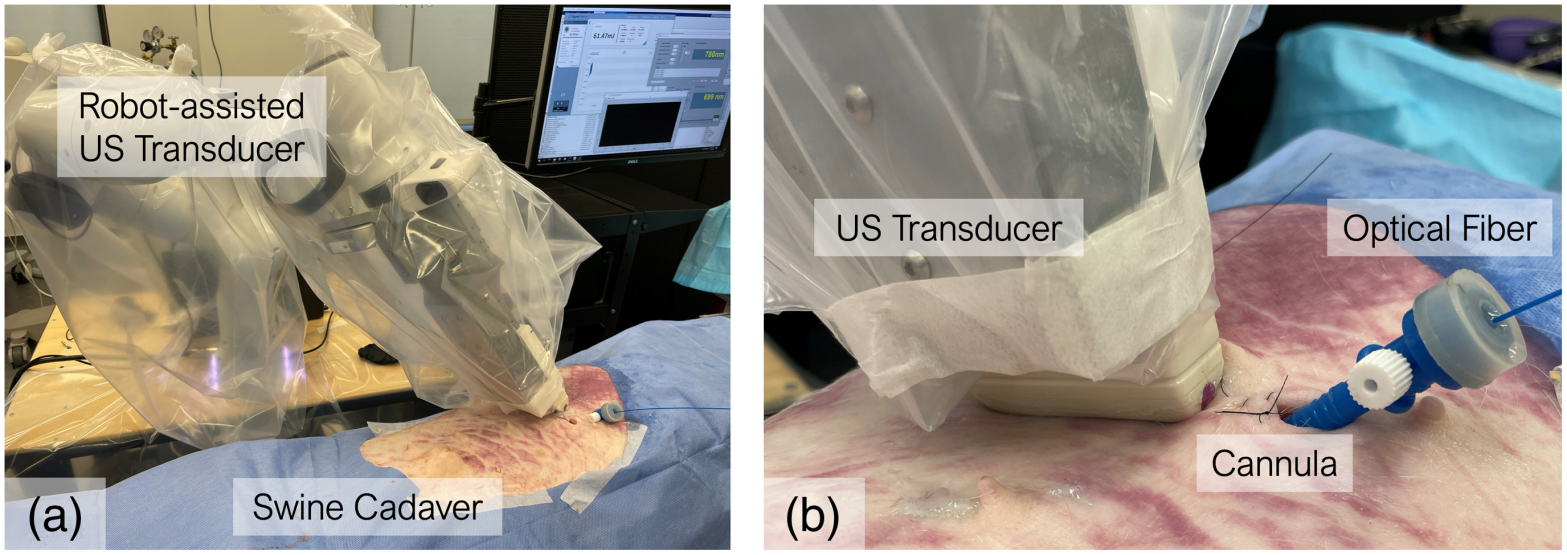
Cadaver study setup: (a) a robot-assisted ultrasound (US) transducer was used to receive US/photoacoustic (PA) signals from the skin surface. (b) The ablation device and optical fiber were inserted into the liver through a cannula.
